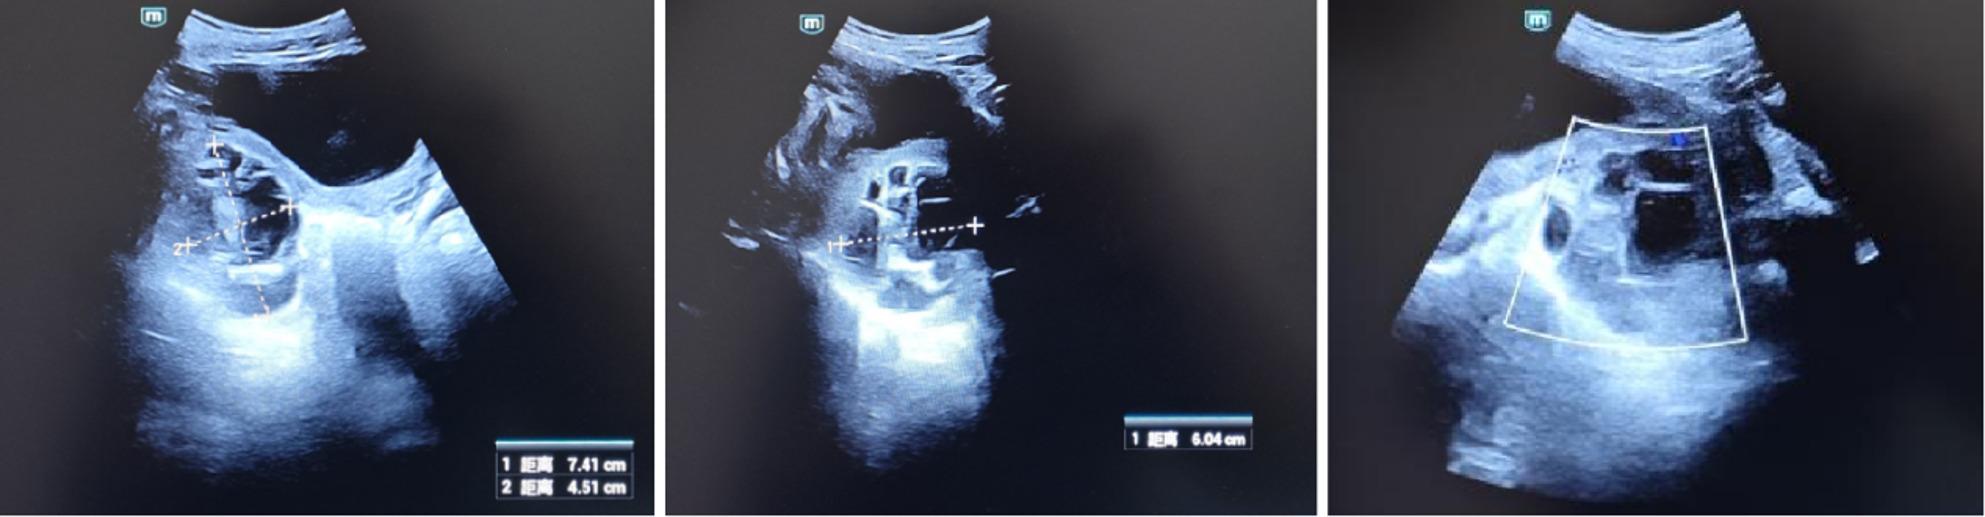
Follow-up transvaginal ultrasound 3 h post-aspiration. The cyst size has reduced to 74 × 60 × 45 mm with persistent internal septations
